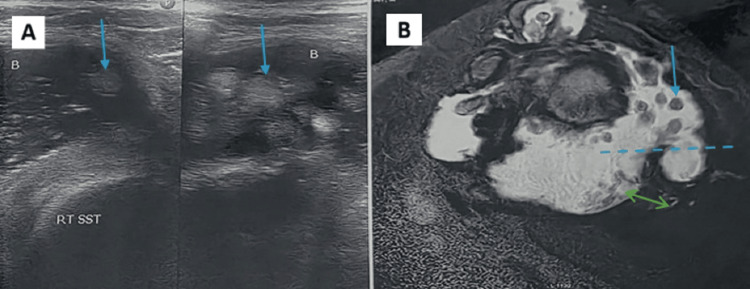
Synovial chondromatosis: (A) USG showing gross joint effusion with multiple echogenic loose bodies (arrow). (B) Coronal PDFS MRI image showing gross joint effusion (dotted arrow) with synovial thickening (double-headed arrow) and multiple hypointense loose bodies (solid arrow) USG, ultrasonography; MRI, magnetic resonance imaging; PDFS, proton density-weighted fat-saturated
